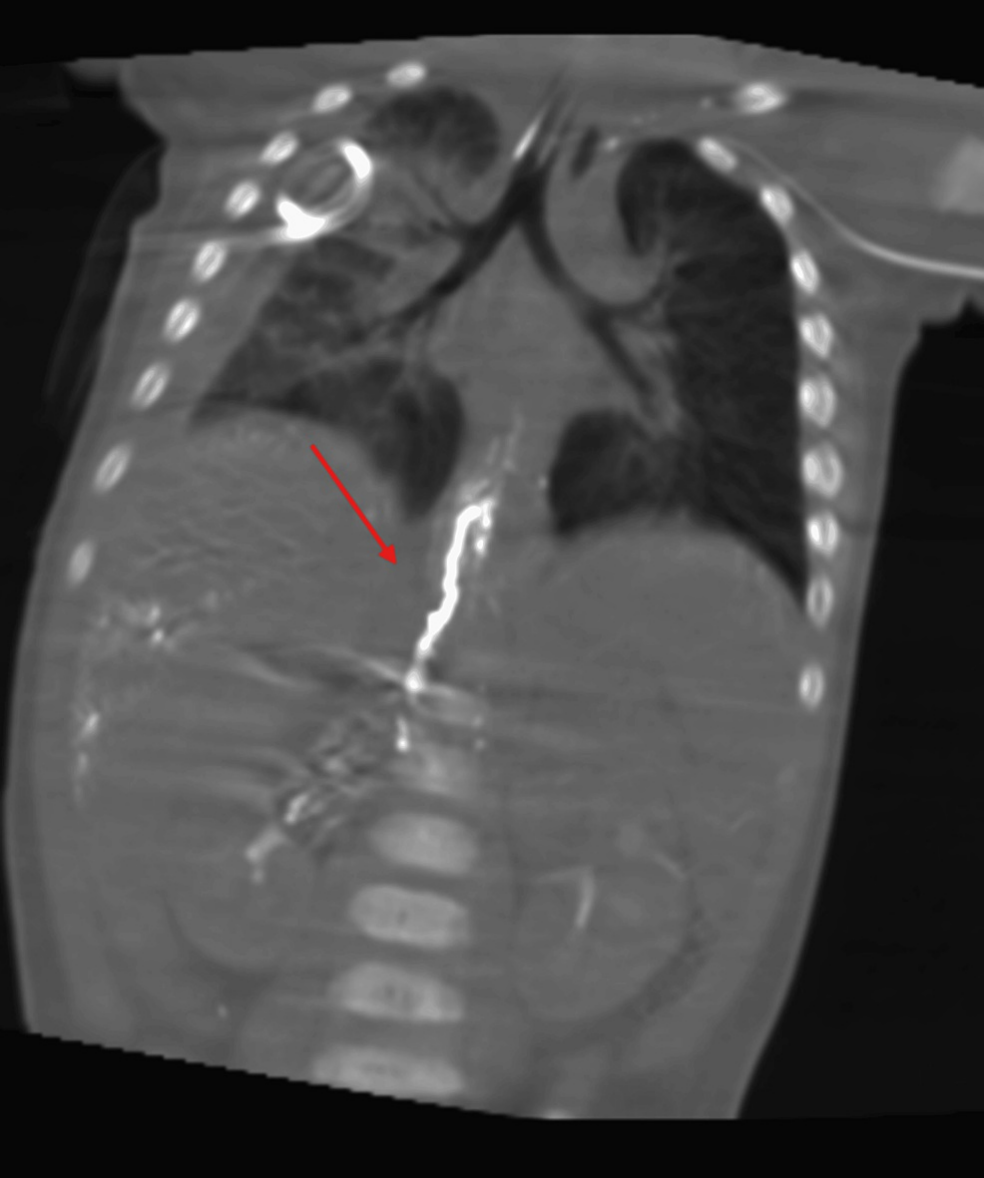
Coronal reformat of cone beam CT showing liquid embolic in the thoracic duct.
